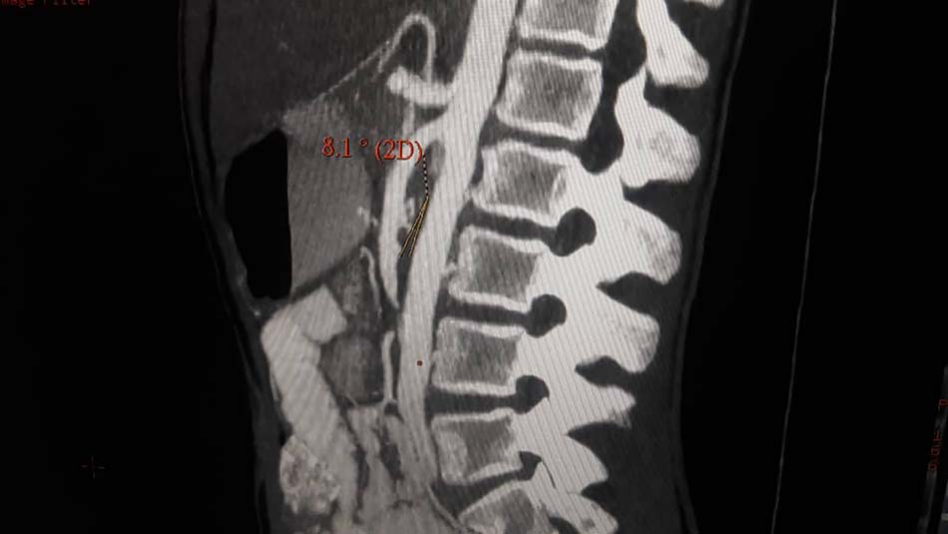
Contrast enhanced CT scan abdomen and pelvis (sagittal view) showing significant reduction of the aortomesenteric angle (measuring 8.1°) and aortomesenteric distance measuring about 7 mm.
